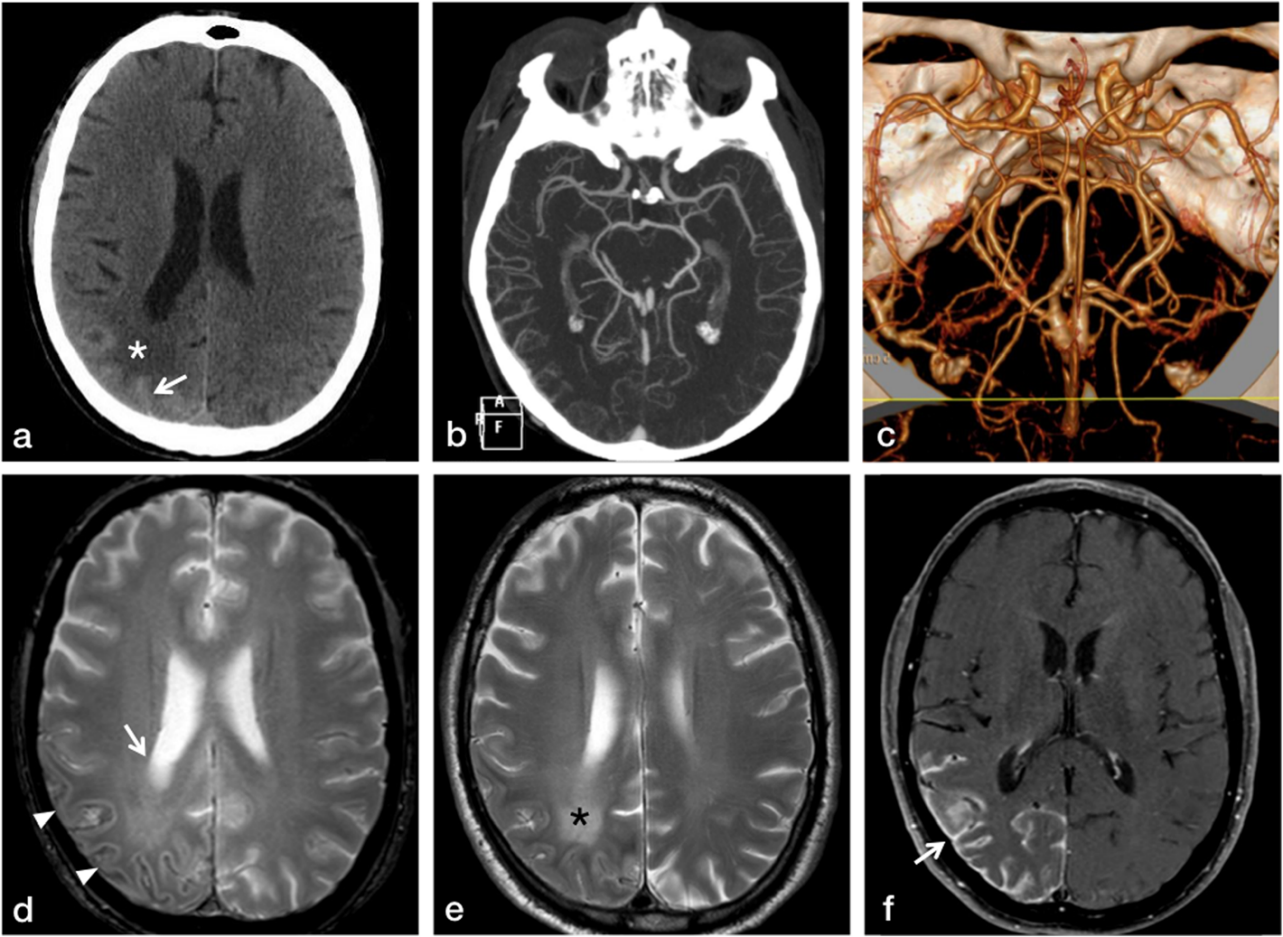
CT (a), CTA (b-c) and MRI brain (d-f). Axial non-enhanced CT (a) shows hyperdense lesion in the right parietal convexity subarachnoid spaces (arrow) associated with hypo-attenuation of subcortical and deep white matter (white asterisk). Maximum Intensity Projection (b) and Volume Rendering (c) from CTA show no significant abnormalities. Axial T2-weighted Gradient Echo (GRE) (d) and Turbo Spin Echo (TSE) images (e) depict curvilinear hypointensity along the right parietal convexity (arrowheads) with hyperintense signal in underlying deep white matter (black asterisk) and subtle enlargement of lateral ventricle (white arrow). Postgadolinium axial Spin Echo (SE) T1-weighted image (f) shows intense serpentine leptomeningeal enhancement along the sulci and gyri of the right parietal lobe (white arrow)
